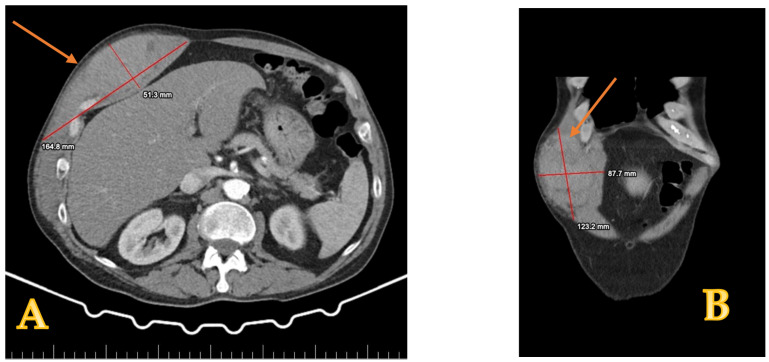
CT scan showing an abdominal mass with an elliptical shape, located in the right rectus sheath, being 123 × 87 mm in size (orange arrow). The images suggest an organized hematoma that is confined to the abdominal wall. The left image (A) depicts the axial aspect while the right (B) image presents the sagittal aspect of the CT scan.
